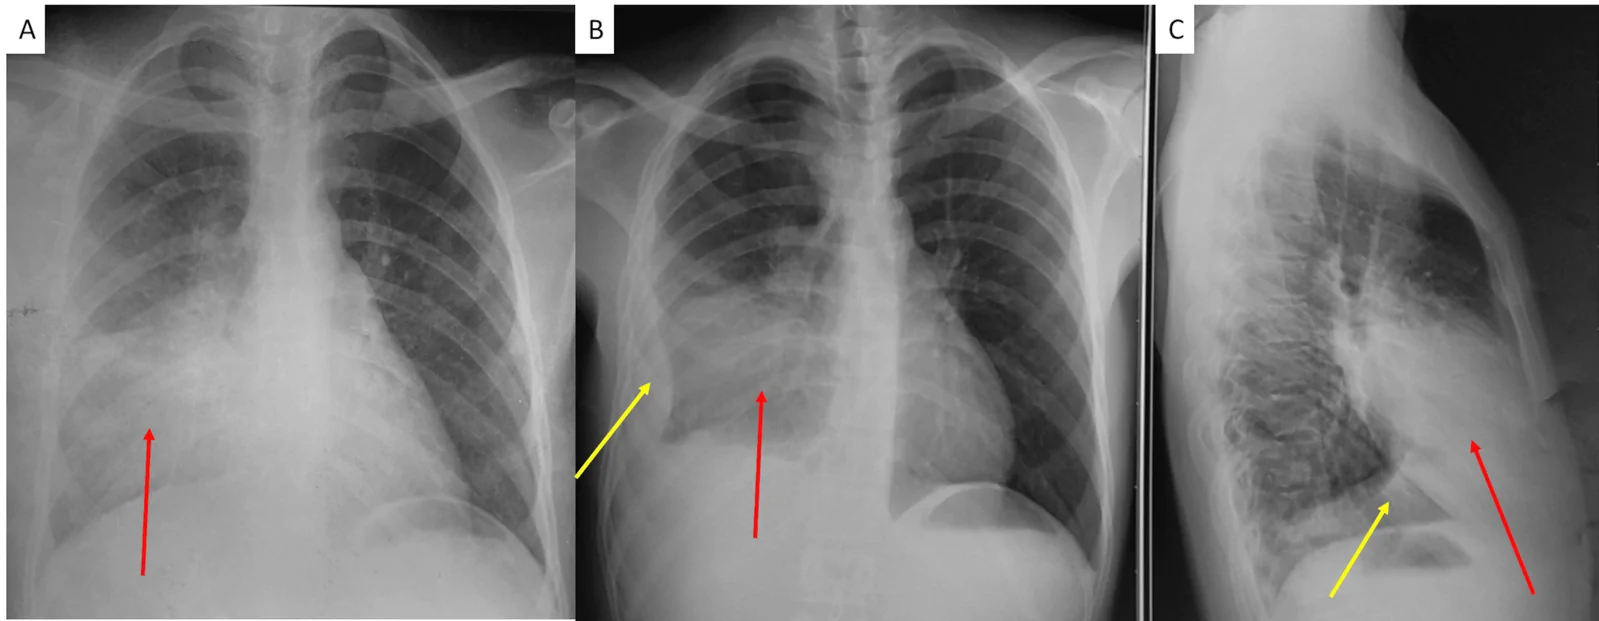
(A) Initial chest radiography showing alveolar opacity in the right middle lobe (red arrow). (B and C) Repeat chest radiography (B: frontal view; C: lateral view) four months later demonstrating persistent right middle lobe consolidation (red arrow) with ipsilateral pleural effusion (yellow arrow).
